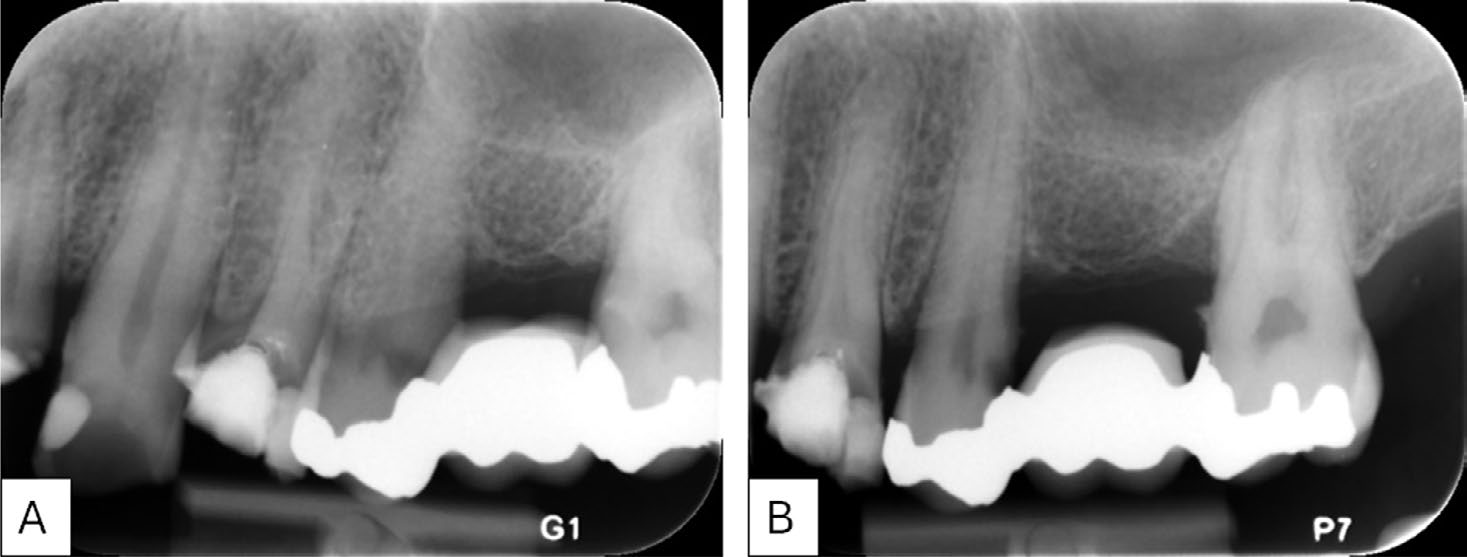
A retake was needed (B) as there was overlap between the teeth and furthermore, the periapical area was not sufficiently displayed (A).
